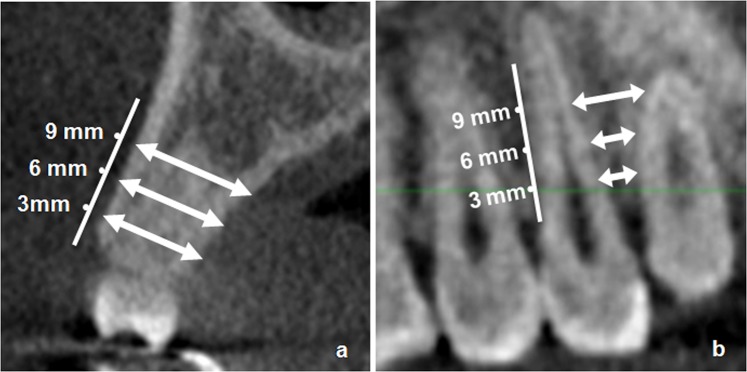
Measurement based on the cross-sectional cone-beam computed tomography images of the available maxillary alveolar bone at 3, 6 and 9 mm of depth from the alveolar ridge. (a) Vestibular-palatine dimension. (b) Mesiodistal dimension.
